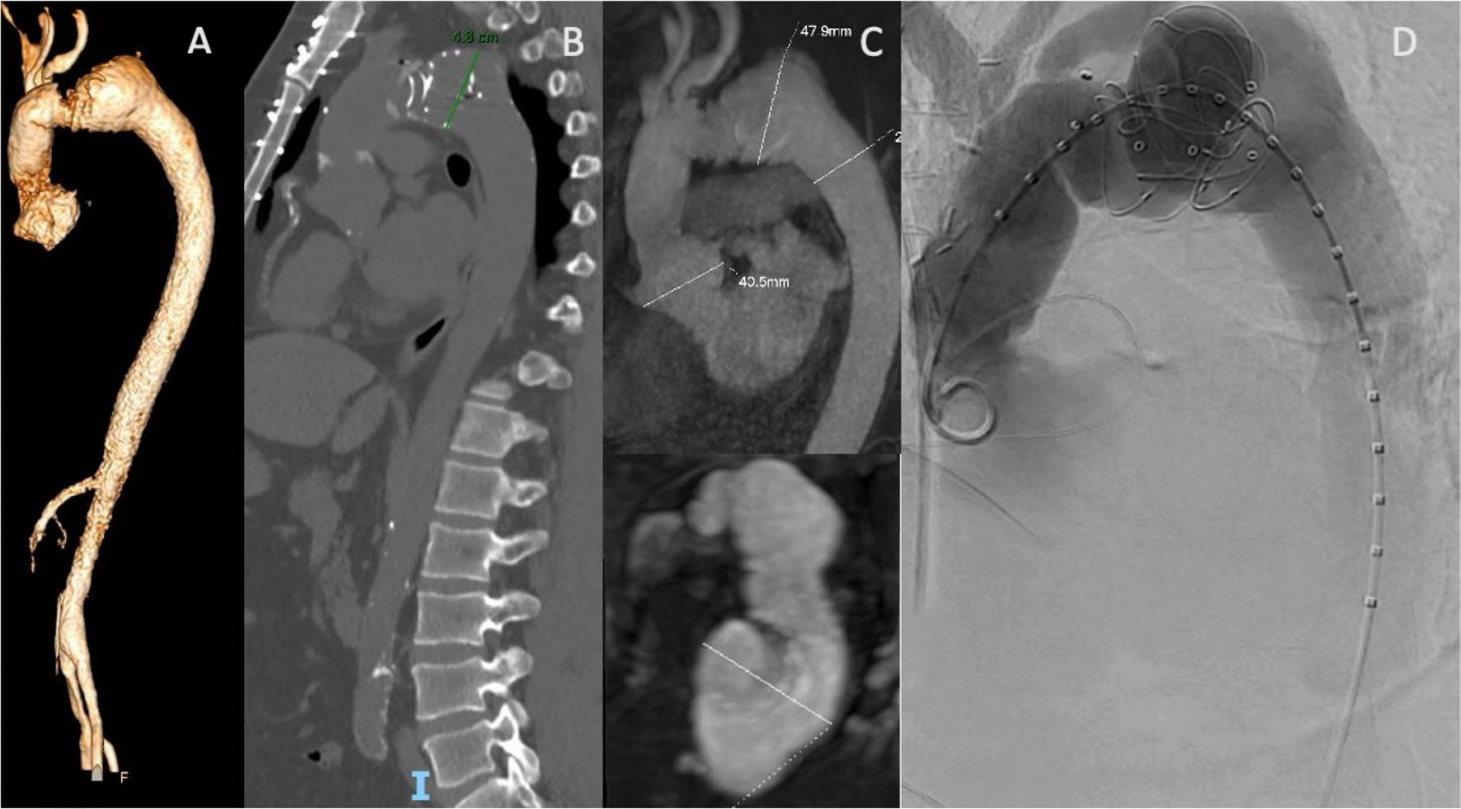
An interesting case of TEVAR extension 6 years after FET. After the patient missed follow-up for 2 years, a control MRI was performed (the patient had recently received a kidney transplantation for Alport syndrome). Malpositioning of the hybrid prosthesis due to proximal migration was found, with the prosthesis not covering the aortic isthmus, which appeared to be dilated (A, C). A CT-scan without contrast confirmed the finding (B). D Angiographic aspect before TEVAR. *TEVAR, thoracic endovascular aortic repair; FET, frozen elephant trunk; MRI, magnetic resonance imaging; CT, computed tomography*
